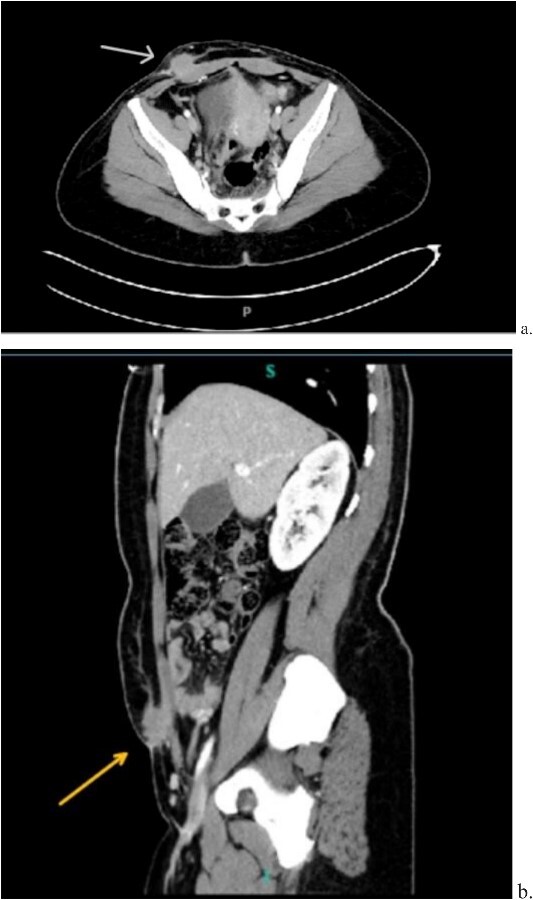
Computed tomography axial (a) and sagittal (b) views of a 2.2 × 2.0 cm enhancing mass on the abdominal wall (arrows).
